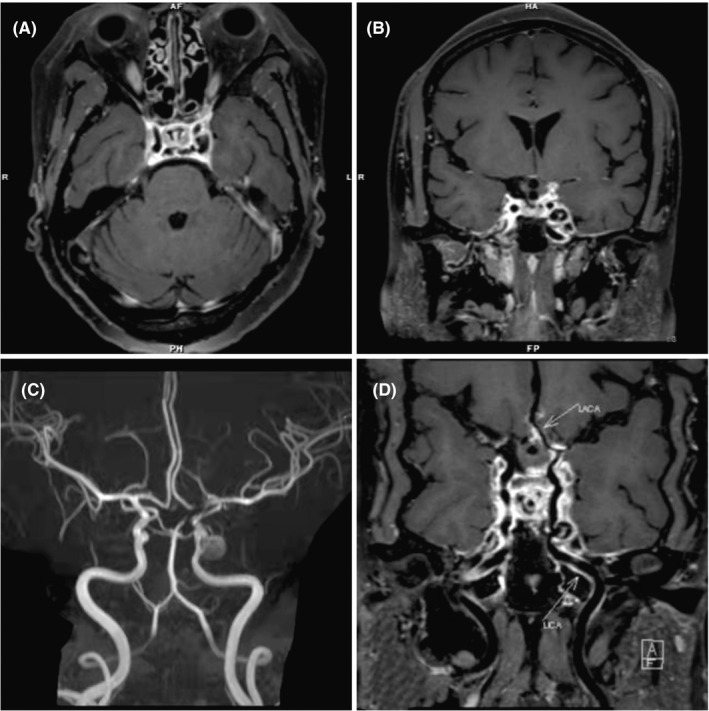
Brain imaging presentations. Axial and coronal T1 contrast‐enhanced MRI scan showing an enlargement of the left cavernous sinus (CS) with an enhancing lesion, an infectious pseudoaneurysm of the Lacerum segment of the left ICA, enhancing lesions encase the left internal carotid artery (ICA), which is narrowed (panel A and B). Three‐dimensional time of flight magnetic resonance angiograms (3D‐TOF MRA) suggests a focal narrowing of the adjacent ICA, the left ICA, left anterior cerebral artery, and left posterior cerebral artery, and Lacerum segment of the left ICA infectious pseudoaneurysm formation (panel C). High‐resolution vessel wall imaging after intravenous contrast injection revealed concentric wall thickening and enhancement of the left ICA, left anterior cerebral artery, and left posterior cerebral artery, compatible with inflammatory vasculopathy (panel D)
